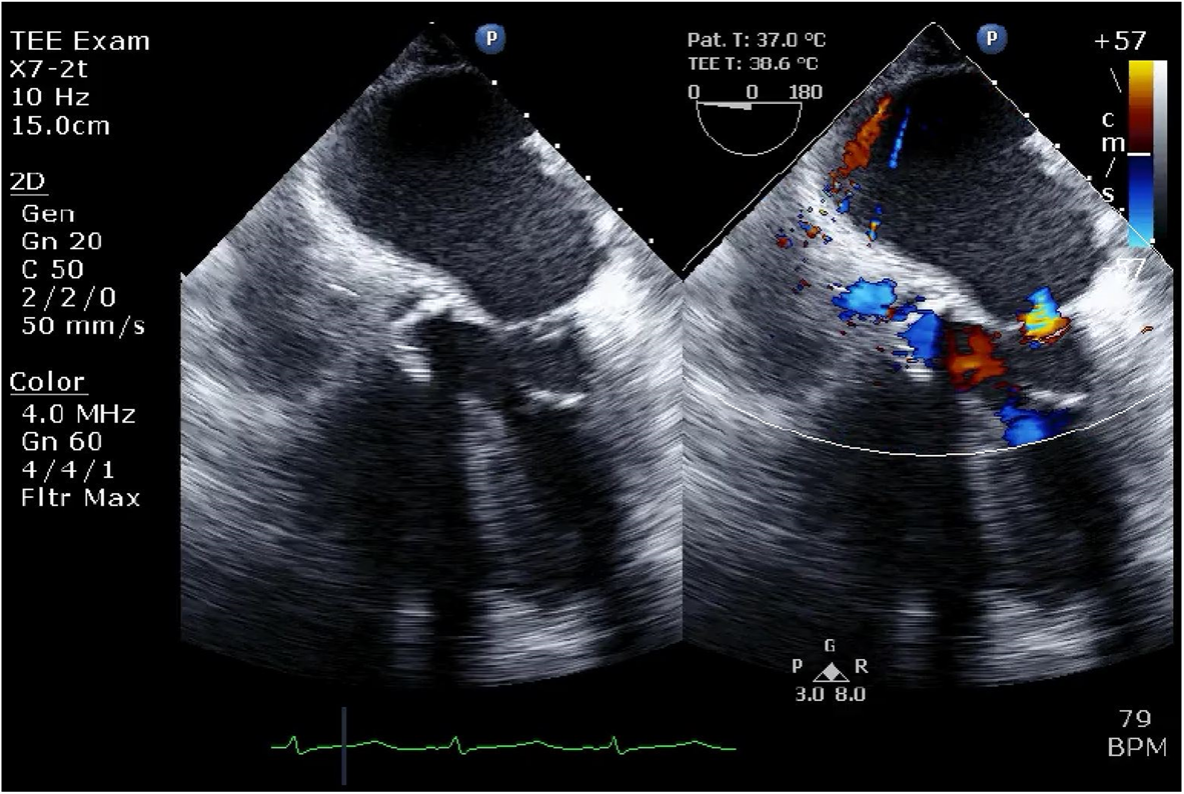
Intraoperative transesophageal echocardiography images after Alfieri stitch was performed, showing a color Doppler image showing mild to moderate residual mitral regurgitation
